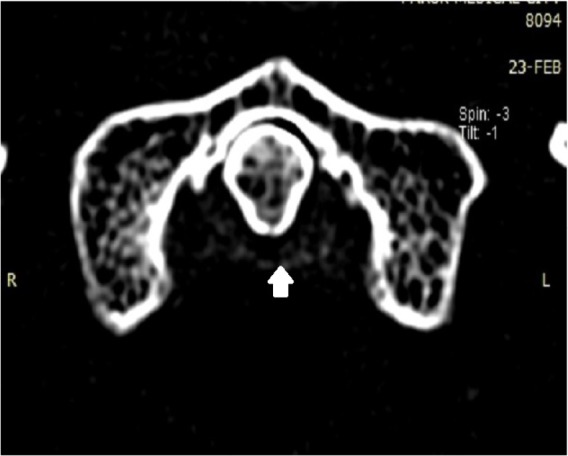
Cervical CT: calcification of the transverse ligament (axial).
